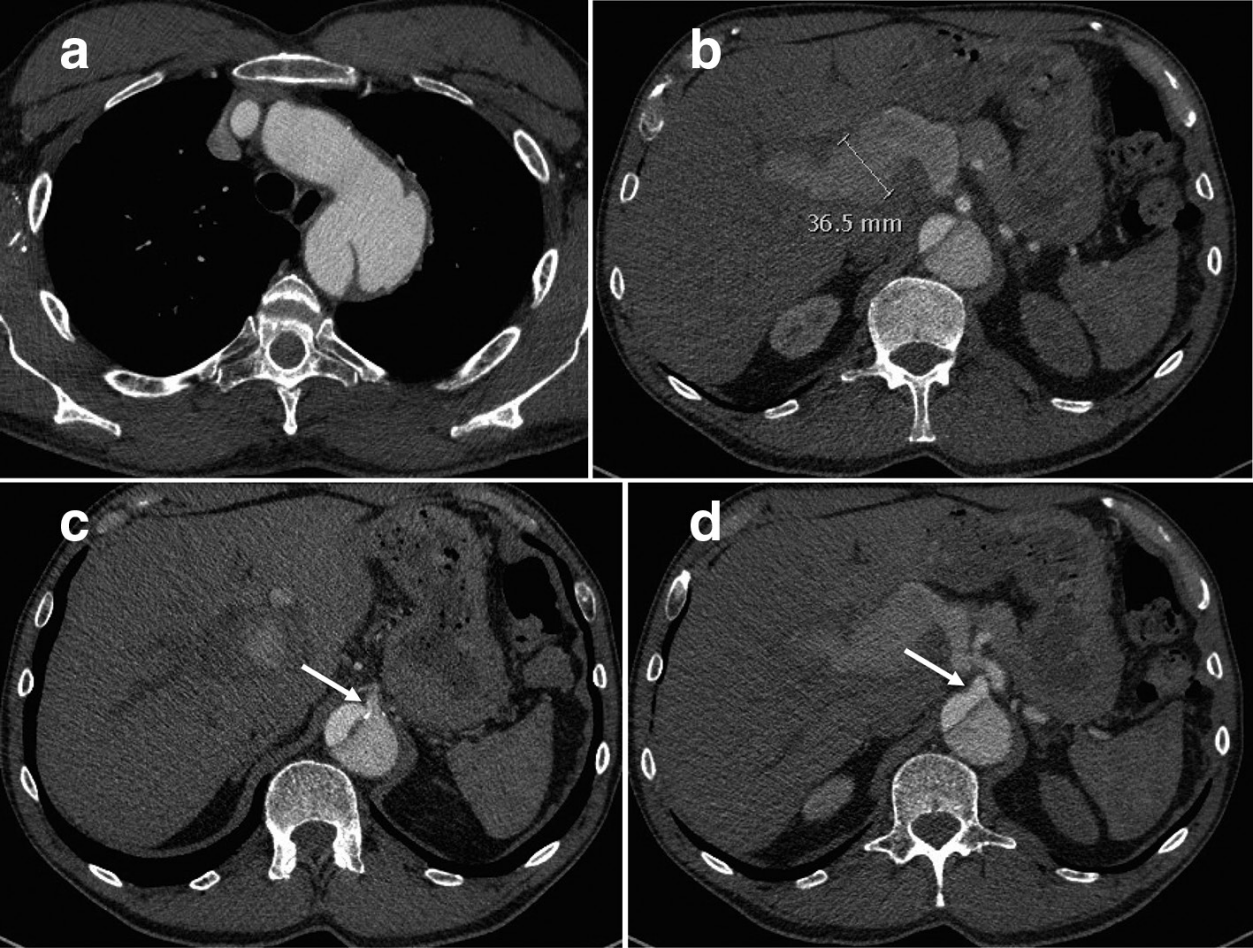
Axial slices from the initial scan – an arterial phase examination performed on a 128-slice Somatom Definition AS + CT scanner (Siemens, Germany) with 90 ml of Omnipaque 300 contrast medium (GE, USA) using bolus-tracking technique; reconstructed into 3 mm slices. All subsequent included scans insubsequent figures were performed with the same parameters unless otherwise indicated. (a) Thoracic slice showing Type B aortic dissection with a wide intimal defect 3 cm distal to the left subclavian artery origin; (b) upper abdominal slice showing a 37 mm fusiform hepatic artery aneurysm, with degree of enhancement similar to the aortic false lumen; (c) shows the aortic intimal flap extending up to the origin of the coeliac artery (arrow - please note this arrow has moved since the figure has been reformatted); (d) shows the SMA origin is supplied by the true lumen (arrow - please note this arrow has moved since the figure has been reformatted). SMA, superior mesenteric artery.
